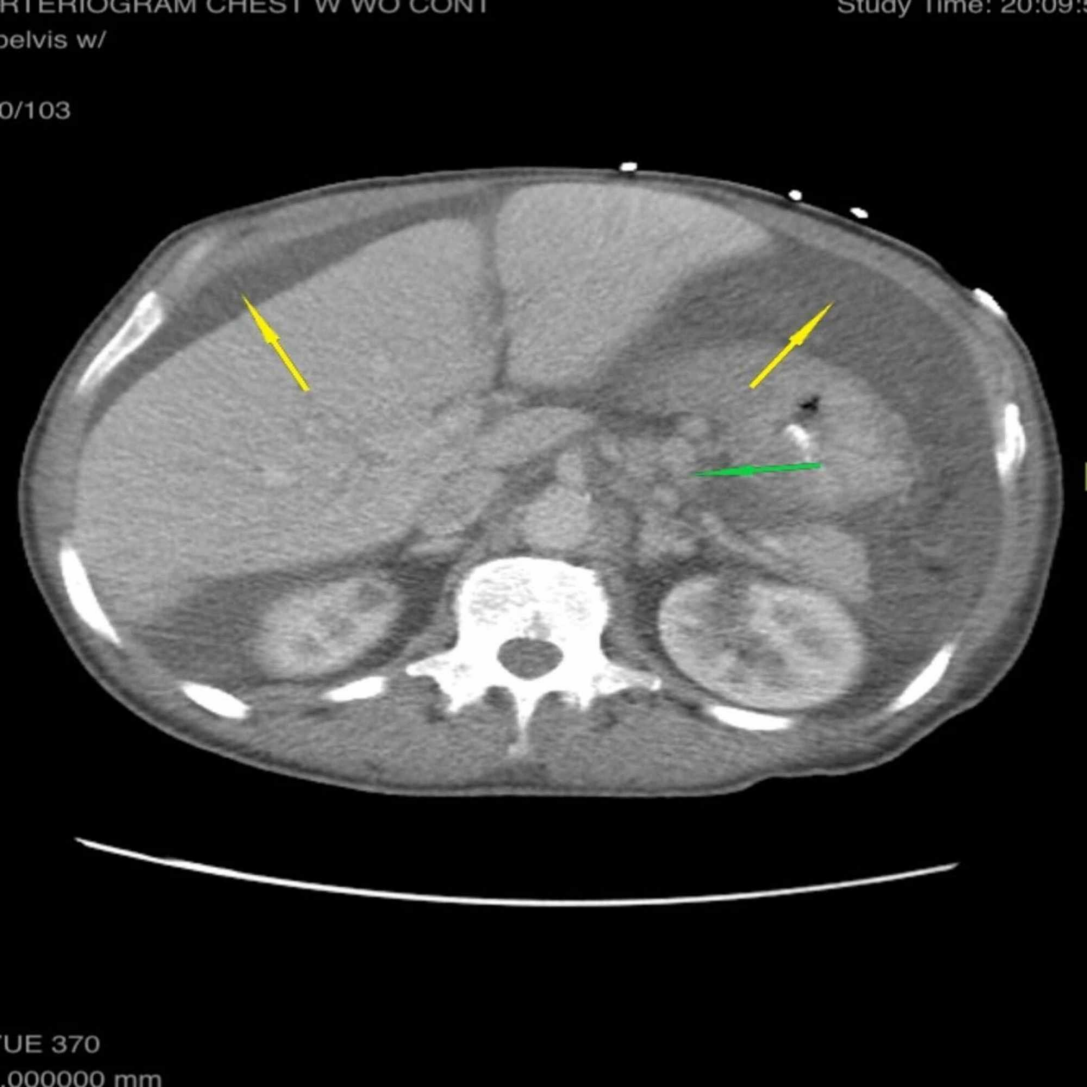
Non-contrast computed tomography of the abdomen in axial view showing gastric fundal varices (green arrow) and large volume ascites (yellow arrows)
